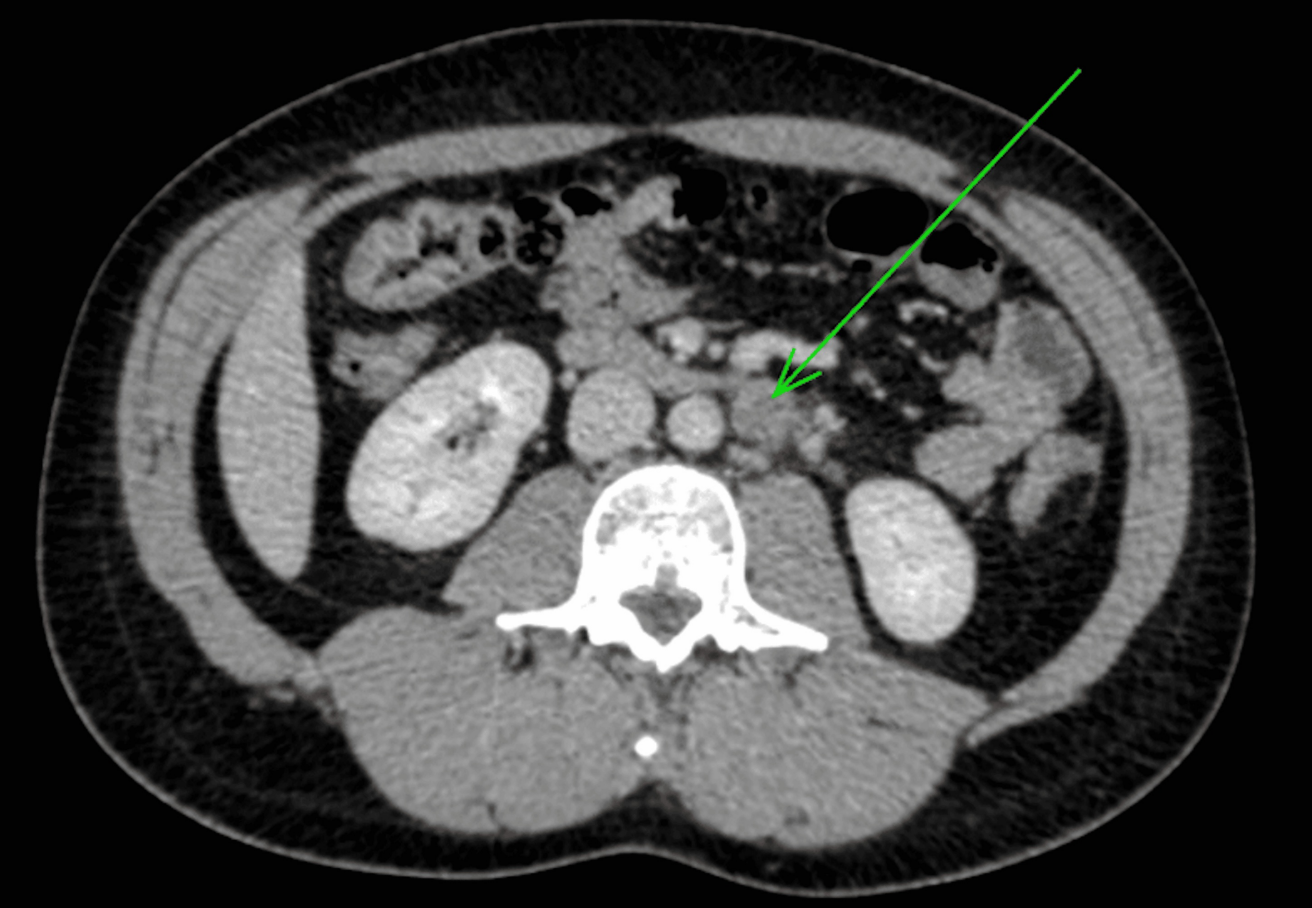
Axial computed tomography scan of the abdomen showing para-aortic lymphadenopathy
